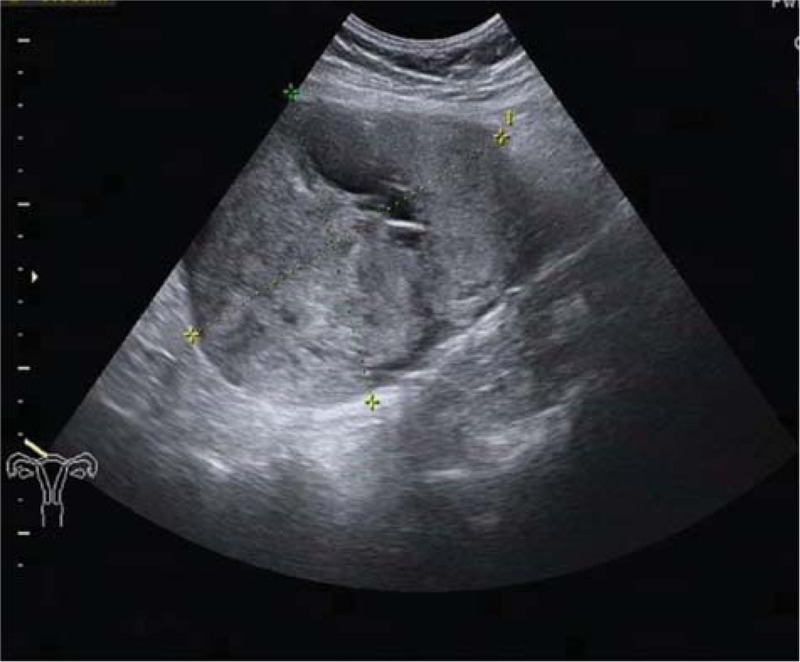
A pelvic mass examined by transvaginal ultrasound. Ultrasonography showed a 12 × 11.4 × 9.8 cm heterogeneous mass behind the uterus, with a cystic dark area of 3.4 × 2.3 cm.
